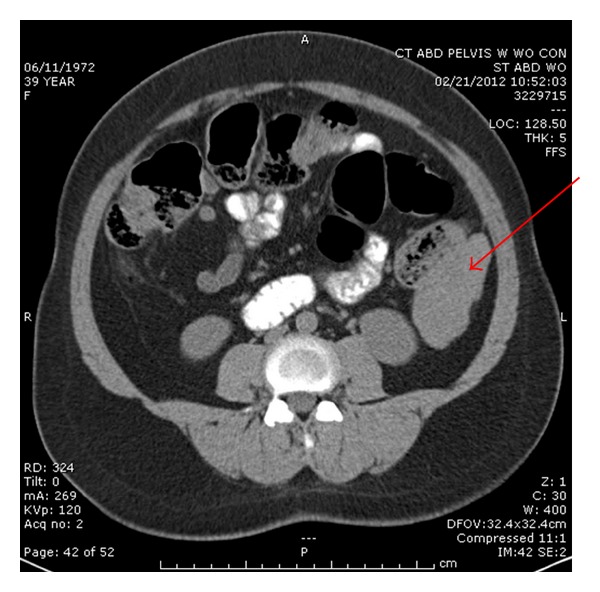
CT scan of the left upper abdominal mass with heterogeneity, adjacent to the descending colon.
